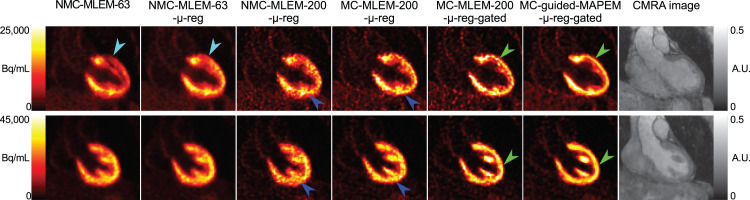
Reconstructed images for each comparative method for 2 representative oncology patients (1 per row). Cyan arrows indicate myocardial defect-mimicking attenuation artifact, which is removed by aligning μ-map using CMRA image. Blue arrows highlight improved local contrast when using motion-corrected PET image reconstruction. Green arrows show reduced noise and improved sharpness when combining motion compensation with MRI-guided PET image reconstruction.
